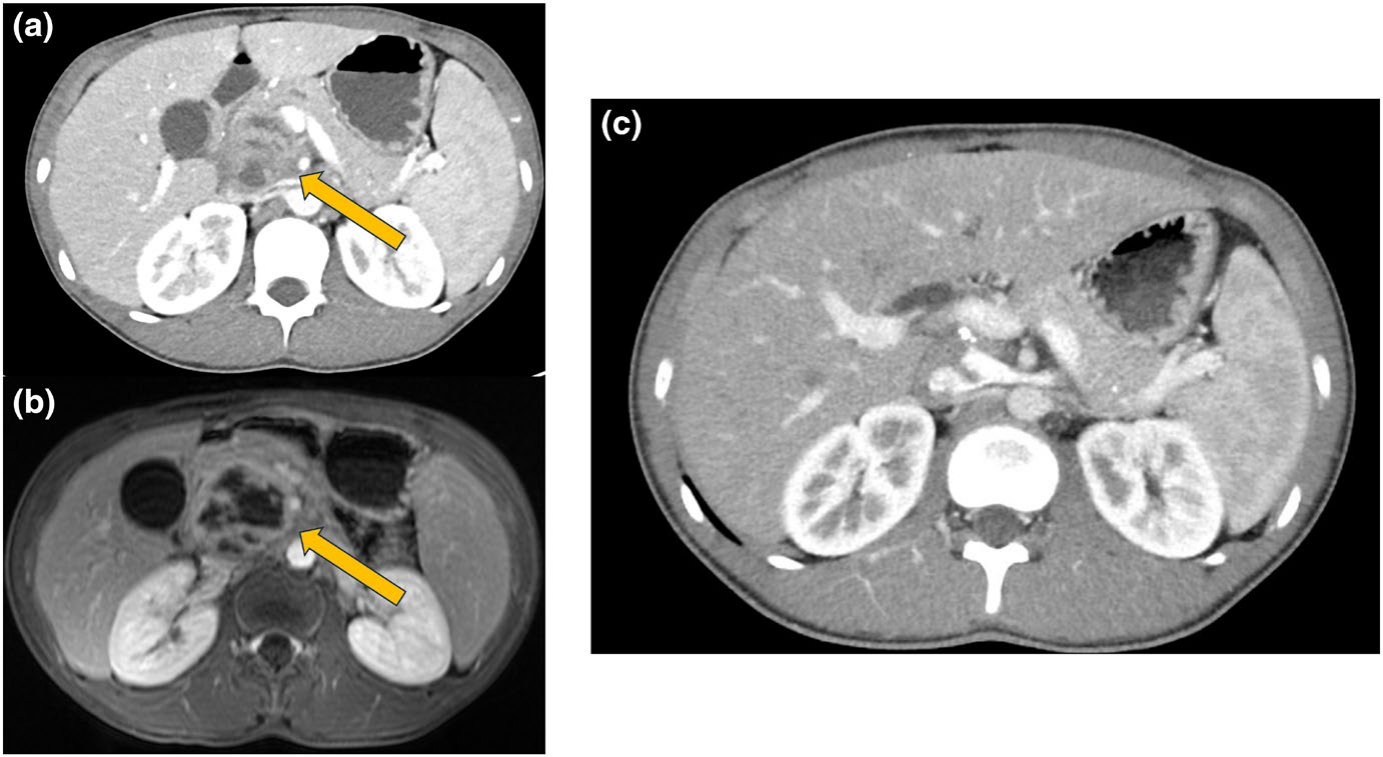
(a) Abdominal contrast‐enhanced CT and (b) T1‐weighted abdominal contrast‐enhanced MRI identified a 6‐cm mass lesion, with degeneration and calcification extending from the pancreatic head to the tail (arrows), along with enlarged periaortic lymph nodes. (c) After treatment, abdominal contrast‐enhanced CT revealed the disappearance of the mass lesion.
